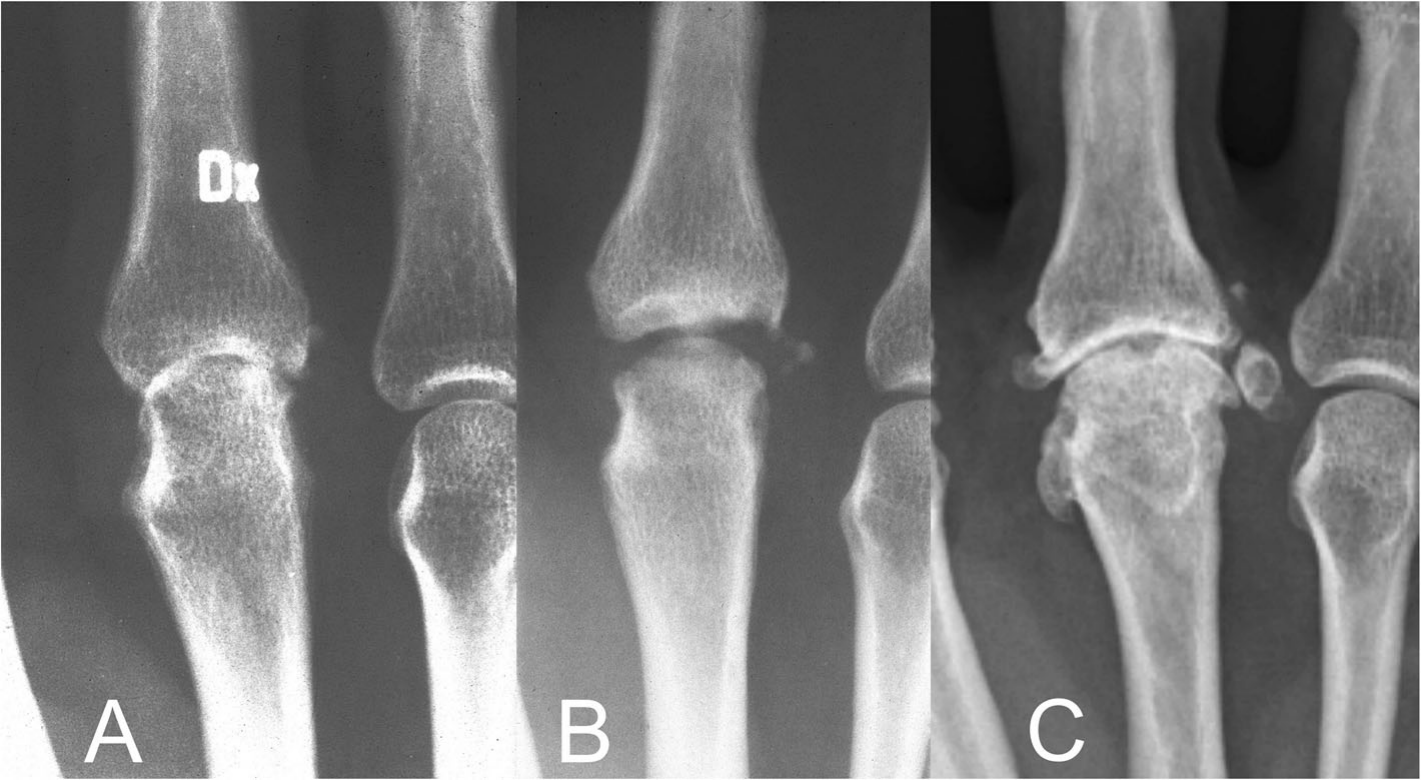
Radiographs (AP view) of the third metacarpophalangeal joint in the primary case from 1974 (case 9) before (a), 6 months after (b), and 40 years after (c) perichondrial reconstruction of the joint. Reproduced with permission of SAGE Publishing [28]
